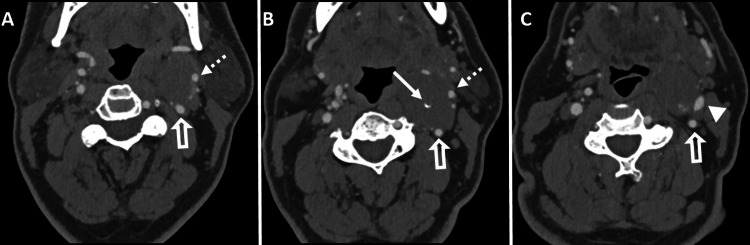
CTA of the neck Axial images were taken after the intravenous injection of 80 mL of iopamidol (Isovue-370; Bracco). The CTA reveals a soft tissue density lesion in the left carotid space with a few punctate calcifications/phleboliths (solid white arrow in B) and mild peripheral enhancement in the arterial phase. There is posterolateral displacement of the left external carotid artery (indicated by dashed arrows in A and B), left internal carotid artery (open white arrows in A-C), and internal jugular vein (solid white arrowhead in C). CTA: Computed tomography angiography.
